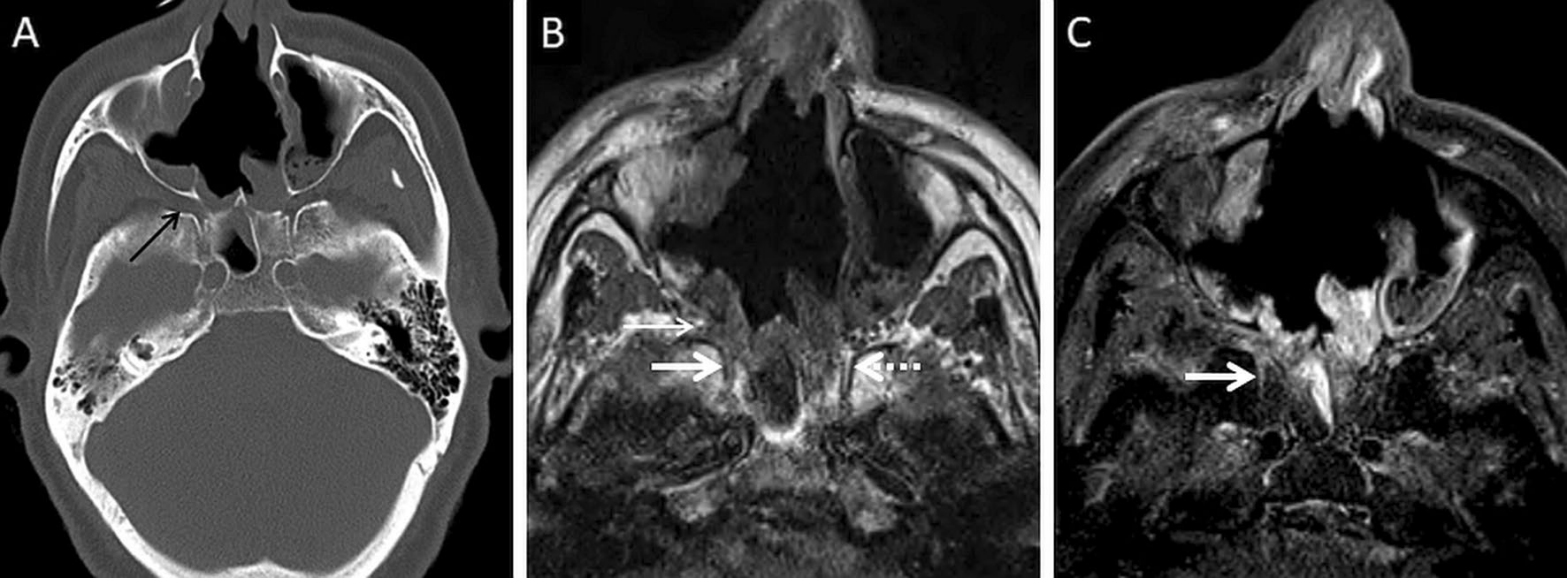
Perineural spread of ROCM detected on post-operative follow-up imaging. Axial CT image (a), obtained using bone algorithm, reveals slight widening of the right PPF (thin arrow). Unenhanced axial T1W image (b) of the same patient confirms soft tissue (thin arrow) within the right PPF. Note the loss of normal fat signal within the right vidian nerve canal (thick arrow), compared to the contralateral side (dotted arrow). CE FS axial T1W image (c) reveals abnormal enhancement of the soft tissue within the right vidian nerve canal (thick arrow). The diagnosis of perineural invasion of MCR was suggested. There was no obvious enhancement along the greater superior petrosal nerve detected at the time of scanning
